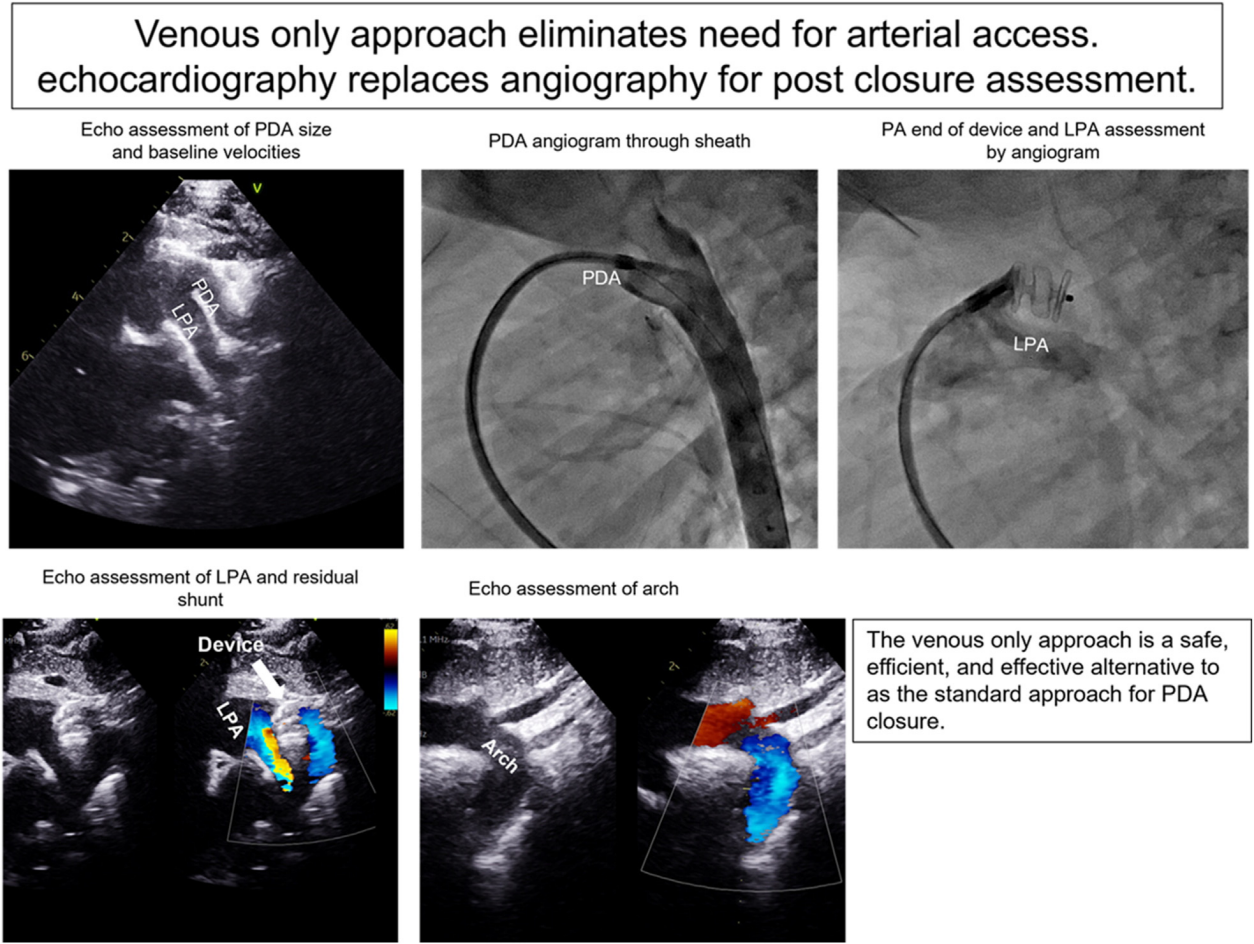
Echocardiography-guided transcatheter patent ductus arteriosus (PDA) closure eliminates the need for arterial access. After device placement, echocardiography replaces angiography for assessing adequacy of closure and to confirm that there is no obstruction to the left pulmonary artery (LPA) or aortic arch before and after release of the device.
